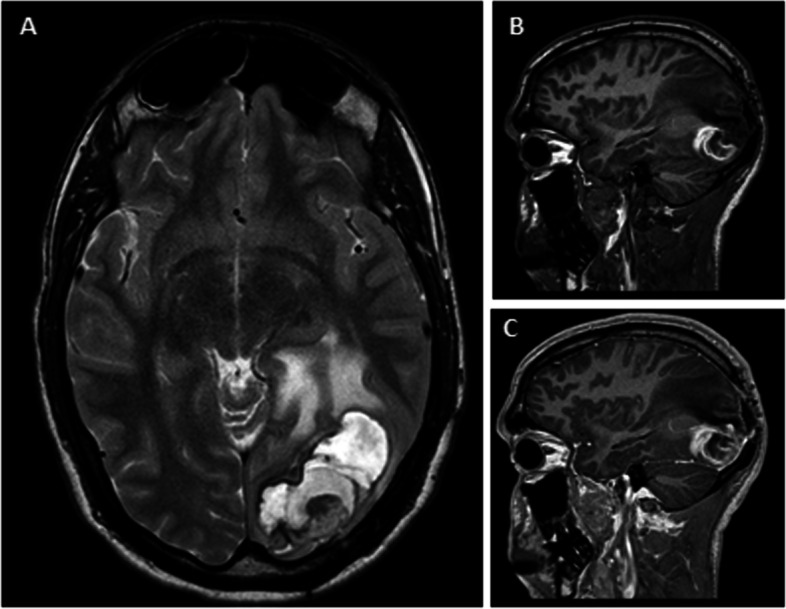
A Axial T2-weighted, B coronal T1-weighted, C coronal gadolinium-enhanced T1-weighted 1 year post-surgical MRI (12 years post-SRS treatment) showing a left occipital chronic encapsulated expanding hematoma (CEEH) with the persistence of the enhancing angiomatous nodule. Note the contrast-enhancing capsule
